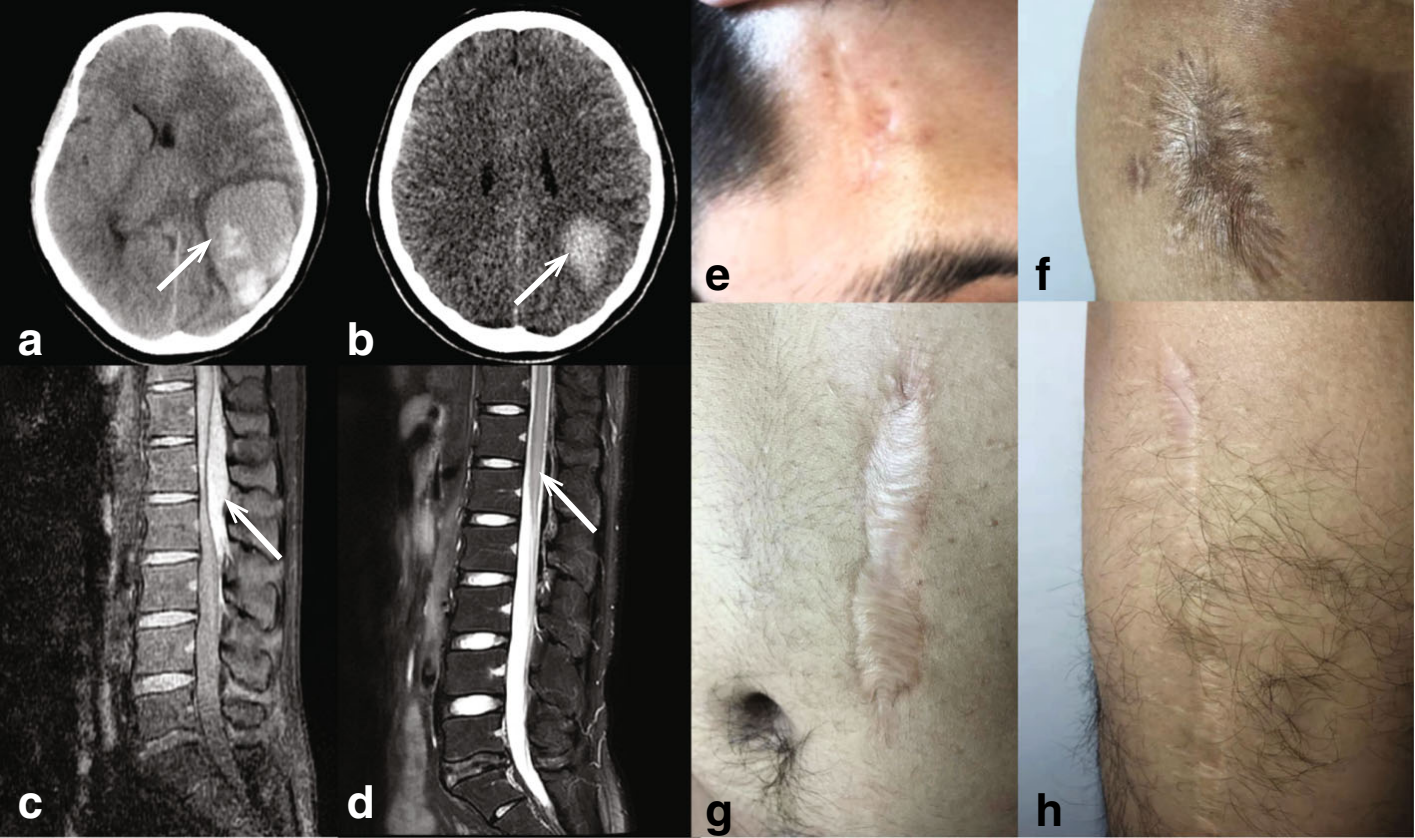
Fetal bleedings and delayed wound healings in probands 1 and 2. a and b Cerebral hemorrhage of the left top occipital lobe at the beginning and 17 days later, respectively shown by computed tomography (CT) in proband 1, indicated by the arrows; c and d Spinal cord hematoma located at T12-L2 at the time of diagnosis and 4 days later, respectively, revealed by magnetic resonance imaging (MRI) in proband 2, indicated by the arrows; e-h Four wounds resulting from delayed wound healing in proband 2. e Forehead; f Right hip; g Left abdomen; h Right thigh
